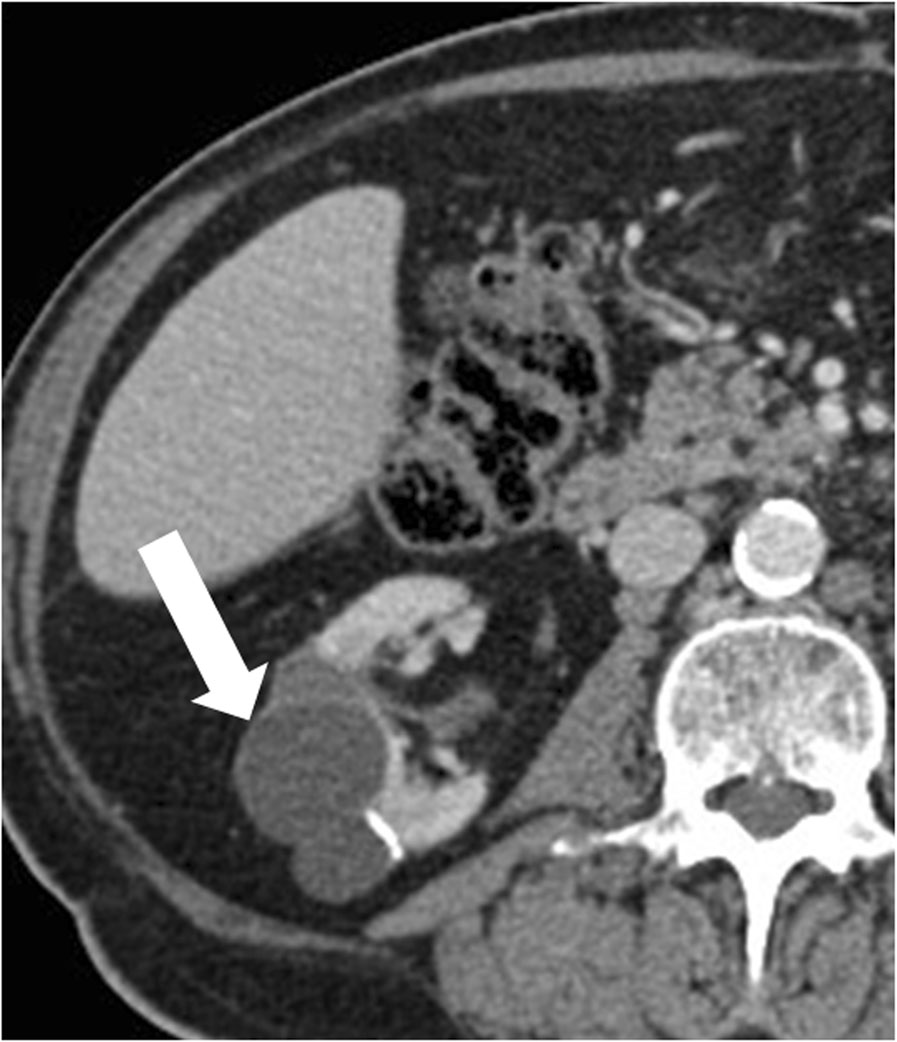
Localized cystic renal disease. Axial contrast-enhanced CT image shows a conglomeration of multiple simple cysts of variable size (arrow) in the right kidney
